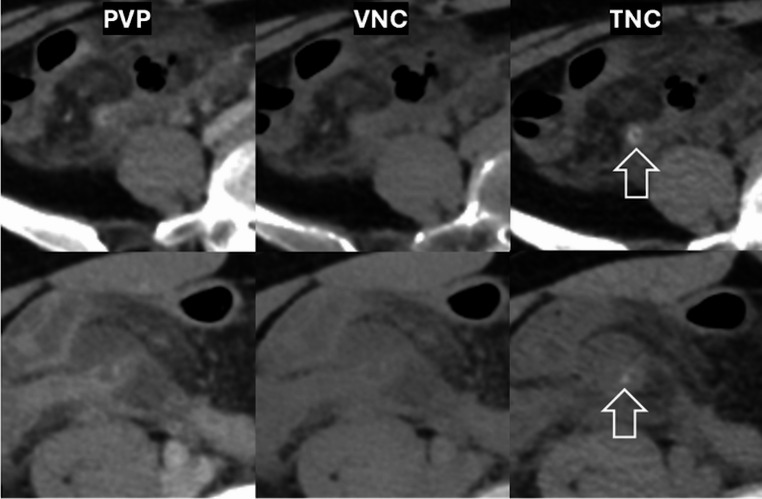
Example of two cases with difficult detection of appendicolith on portovenous phase (PVP) and virtual noncontrast (VNC) dual-energy CT. In the top row, an appendicolith was rather large and clearly hyperattenuating on TNC but it was difficult to appreciate on PVP and VNC. In the bottom row, small size and low attenuation likely make the appendicolith difficult to detect on PVP and VNC. *Arrow* appendicolith
